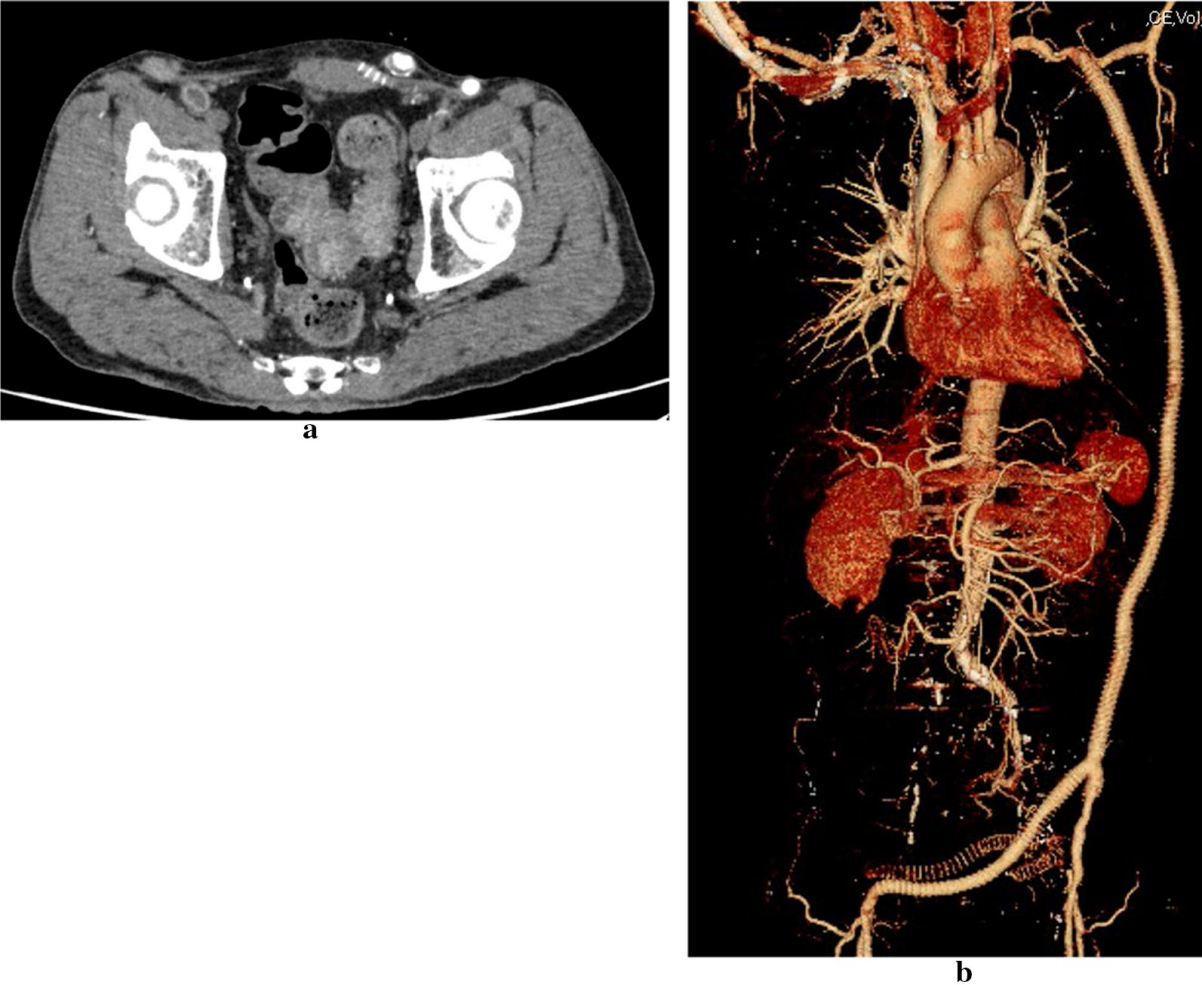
a Preoperative CT. The sigmoid colon had thickened walls and increased concentration of surrounding adipose tissue, but no swollen lymph nodes around the sigmoid colon lesion. b Preoperative blood vessel construction by 3D image analysis software SYNAPSE VINCENT® (Fujifilm, Tokyo)
